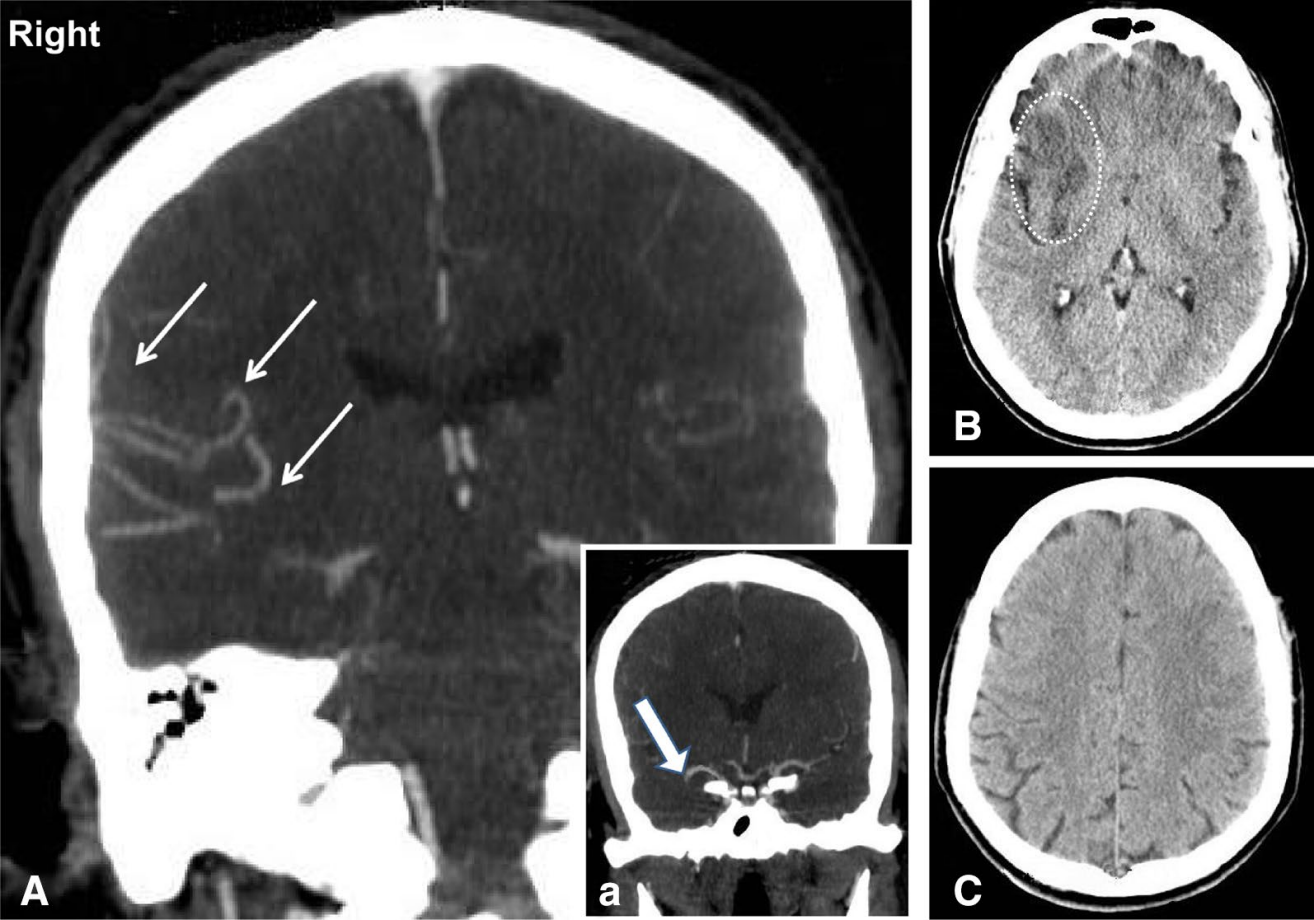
Clinical imaging of cerebral collaterals during acute ischemic stroke using CT-angiography. Collateral vessels [(A) *small arrows*] are visible in the right hemisphere. These vessels have been recruited after acute right MCA occlusion [(a) *large arrows*]. This patient was treated with intravenous thrombolysis and developed a small subcortical lesion (B), while the entire cortical territory was intact (C)
